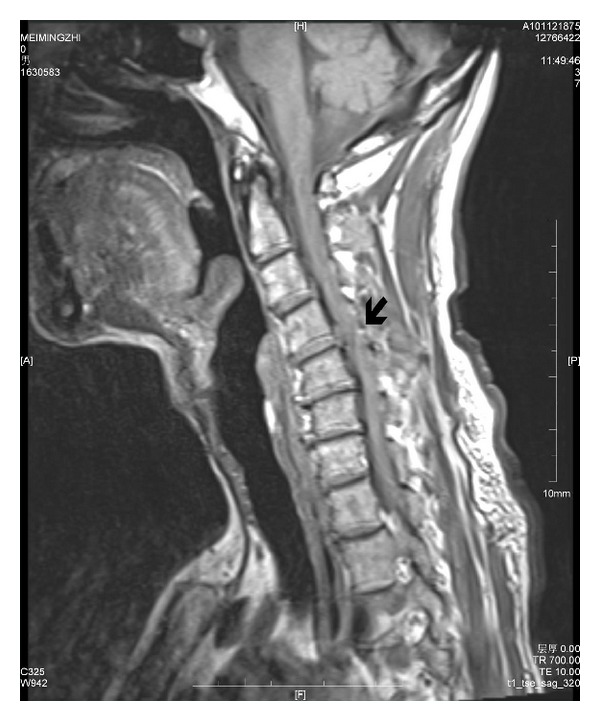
T2-weighted MRI demonstrates disc protrusion at C3-C4, C4-C5, and C5-C6 and corresponding spinal canal stenosis (preoperative MRI). Arrow shows the lesion at the C4-5 level before the operation.
